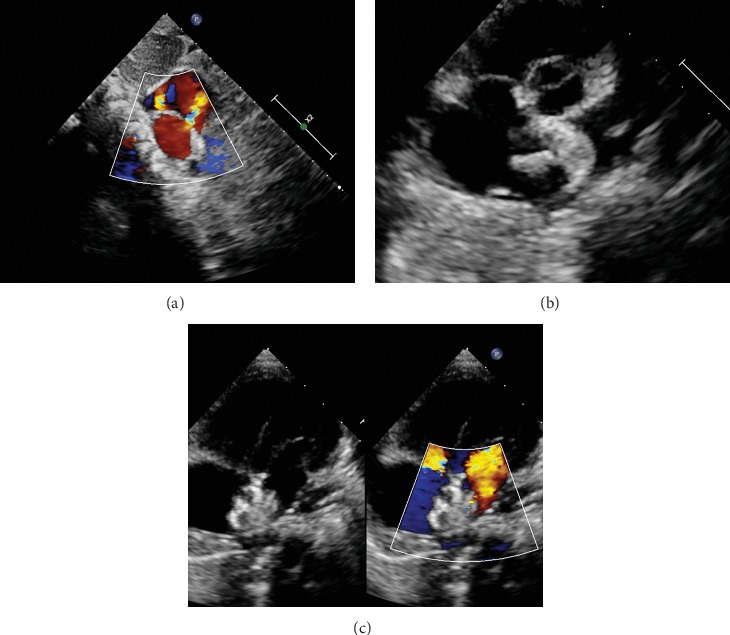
Percutaneous closure of multiple ASDs under TTE guidance. (a) Multiple ASDs image as displayed in subcostal view. (b) The left disc was released (parasternal short-axis view). (c) The ASDs were closed (four-chamber view).
